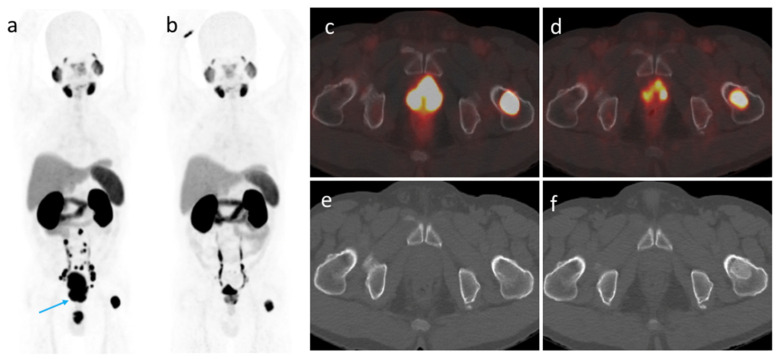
A 60-year-old patient with metastatic prostate cancer (clinical stage IVB, PSA 11.54 ng/mL, Gleason score 5 + 4). (a) A maximum intensity projection obtained before treatment revealed the prostate tumor (blue arrow) accompanied by urine contamination. (b) A second scan after three months of leuprorelin therapy showed a significant response to treatment. (c) and (d) Axial fused PET/CT images revealed a decreased prostate tumor metabolic volume and a reduced tracer uptake. The SUVmax of a left femoral metastatic lesion decreased from 70.41 to 20.32. (e) and (f) A second morphological imaging examination revealed a bony sclerotic change of the left femoral metastatic lesion, which was unexpected based on PET/CT findings.
